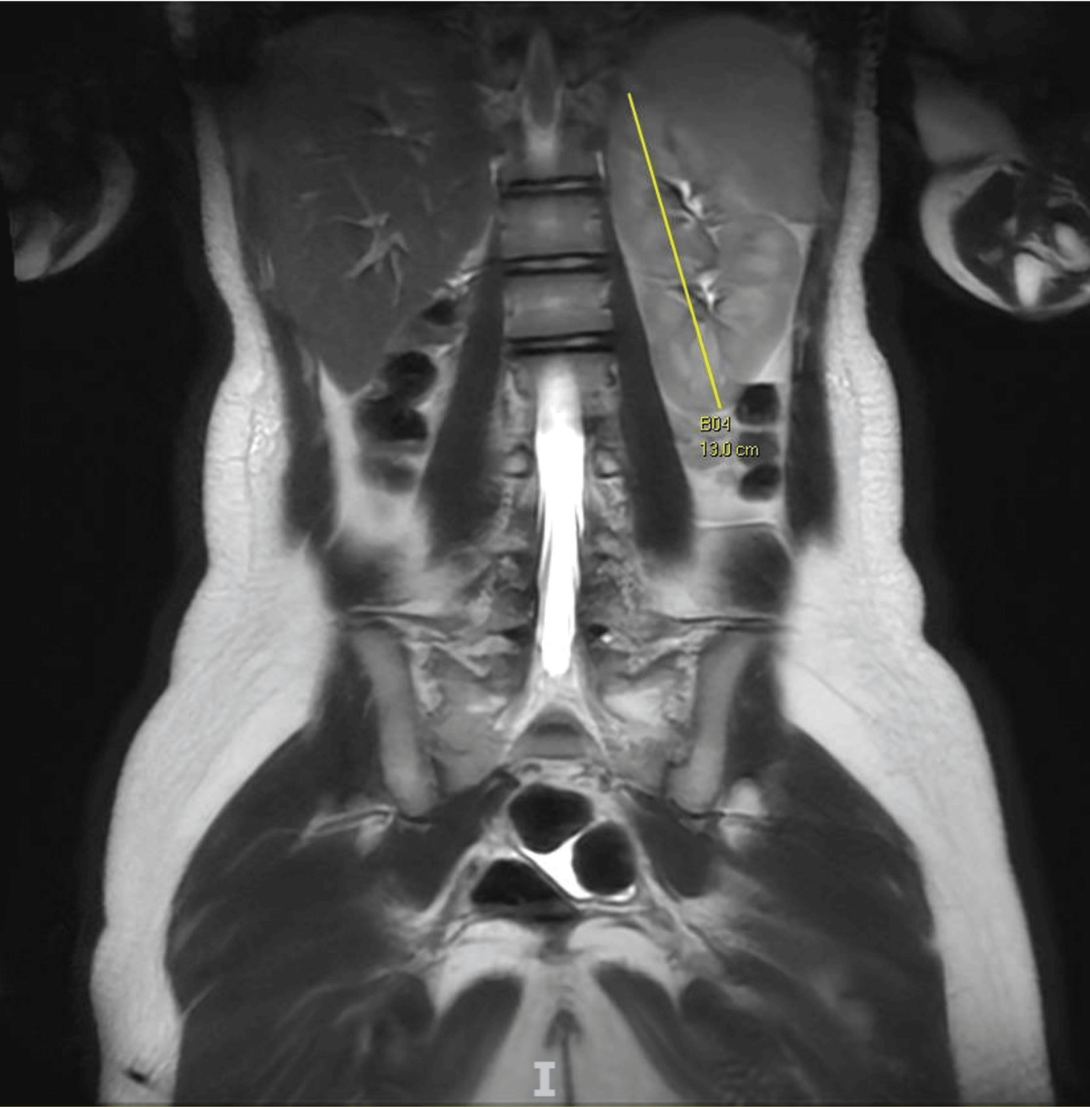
Coronal view on MRI showing right renal agenesis and left solitary kidney MRI: magnetic resonance imaging
